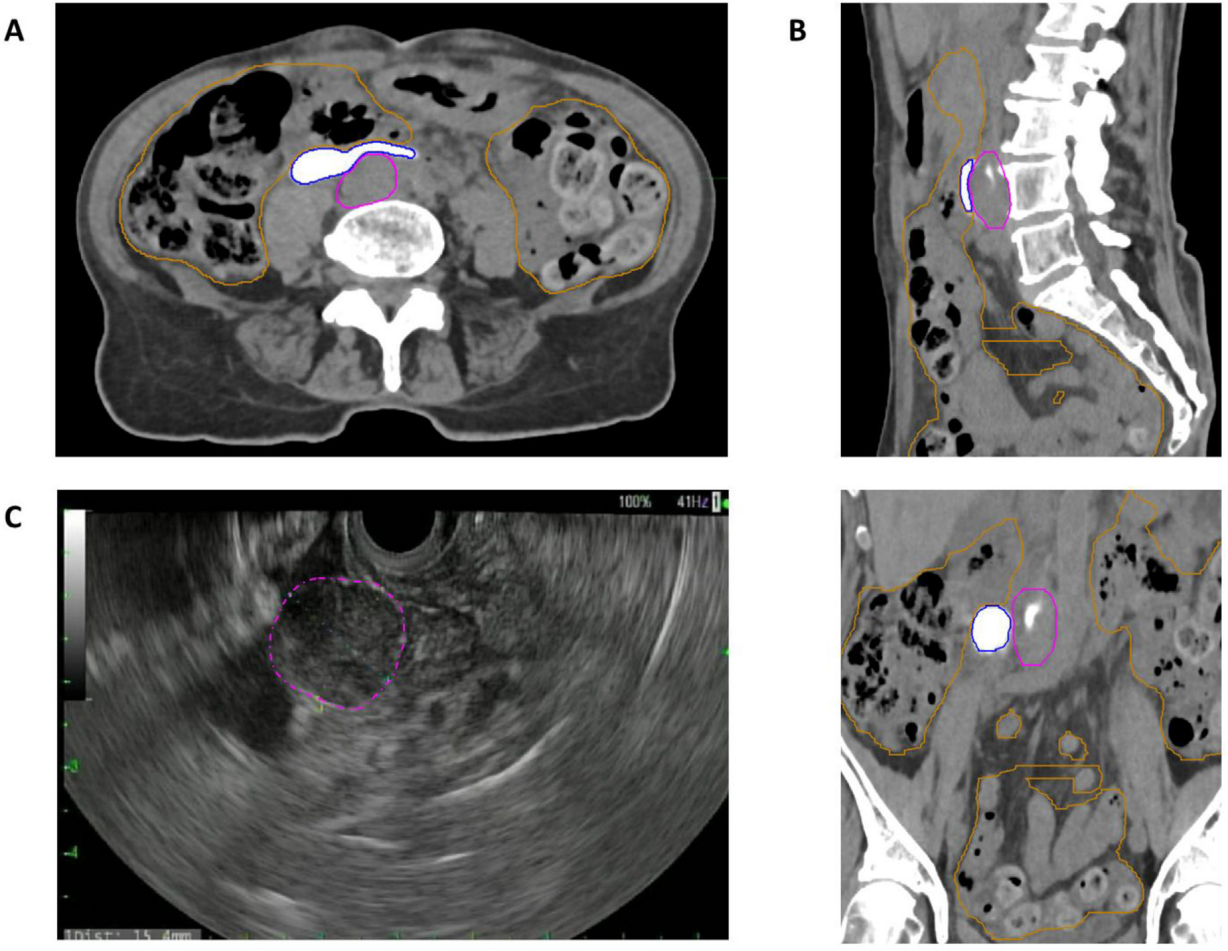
Multimodality imaging demonstrating successful endoscopic placement of a hydrogel spacer (SpaceOAR) anterior to aortocaval lymph node (LN1) to physically displace adjacent bowel structures. (A) Axial, (B) sagittal and coronal noncontrast computed tomography (CT) images confirm the radio-opaque spacer (outlined in blue) positioned between the lymph node (outlined in pink) and adjacent bowel loops (outlined in orange). The hydrogel spacer measured 9.27 cc (dimensions: 5.3 × 3.0 × 1.3 cm). (C) Endoscopic ultrasound (EUS) image obtained during spacer injection shows LN1 (dashed pink contour) prior to hydrogel instillation.
